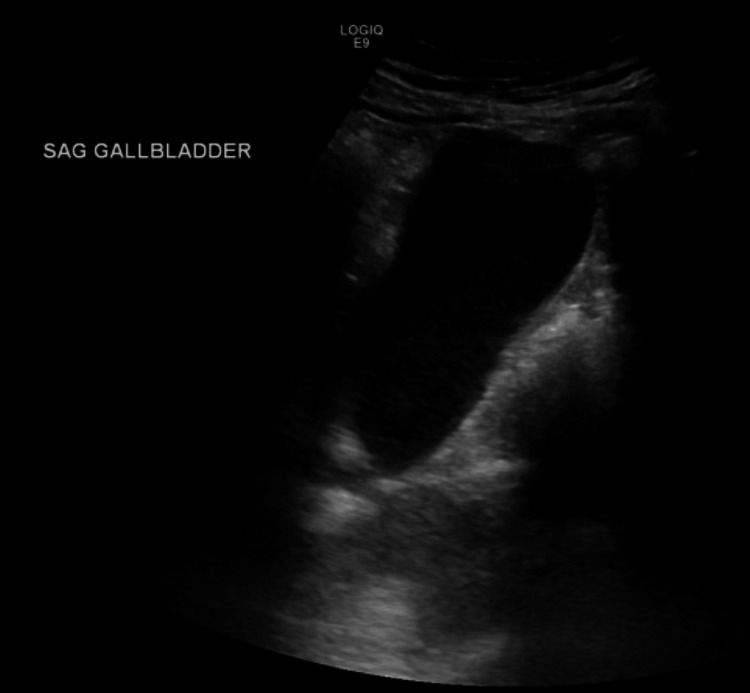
Right upper quadrant ultrasound demonstrating a distended gallbladder without gallstones or inflammatory changes.
